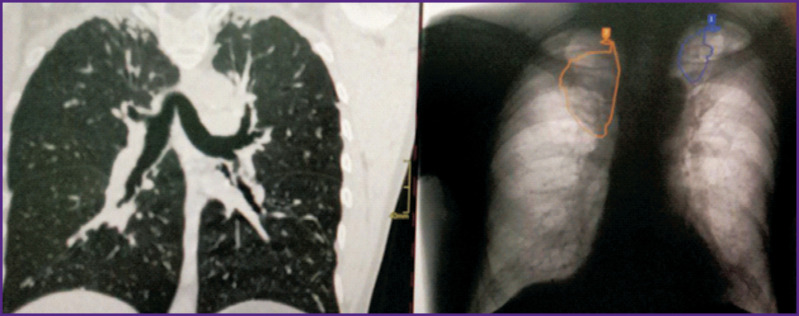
Fluorography stream processing using PhthisisBioMed service (*right*) and CT scan results (*left*)
